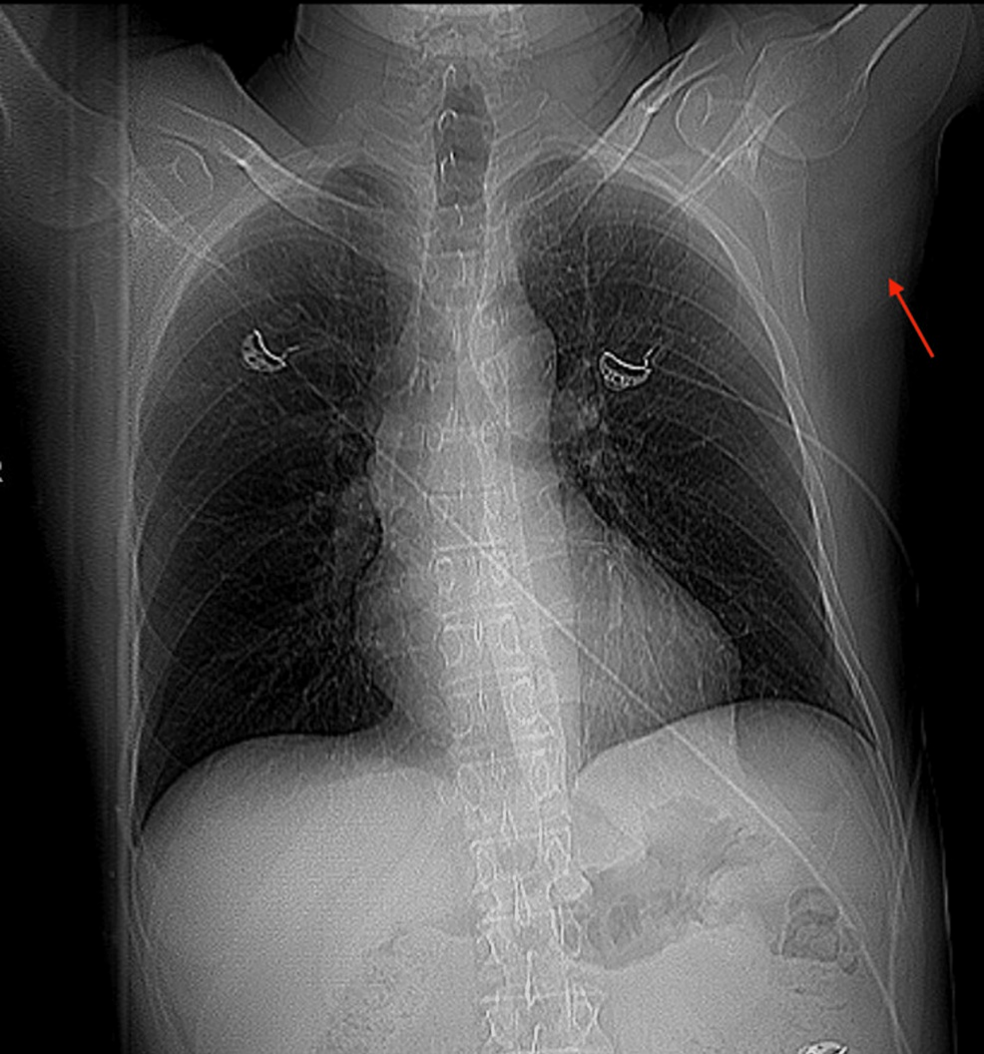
CT of the chest Nonspecific heterogenous attenuation/soft tissue edema lateral to the left pectoralis major with a few internal nonenlarged prominent lymph nodes with obscuration of the left anterior subscapularis muscle, as indicated by the red arrow.
